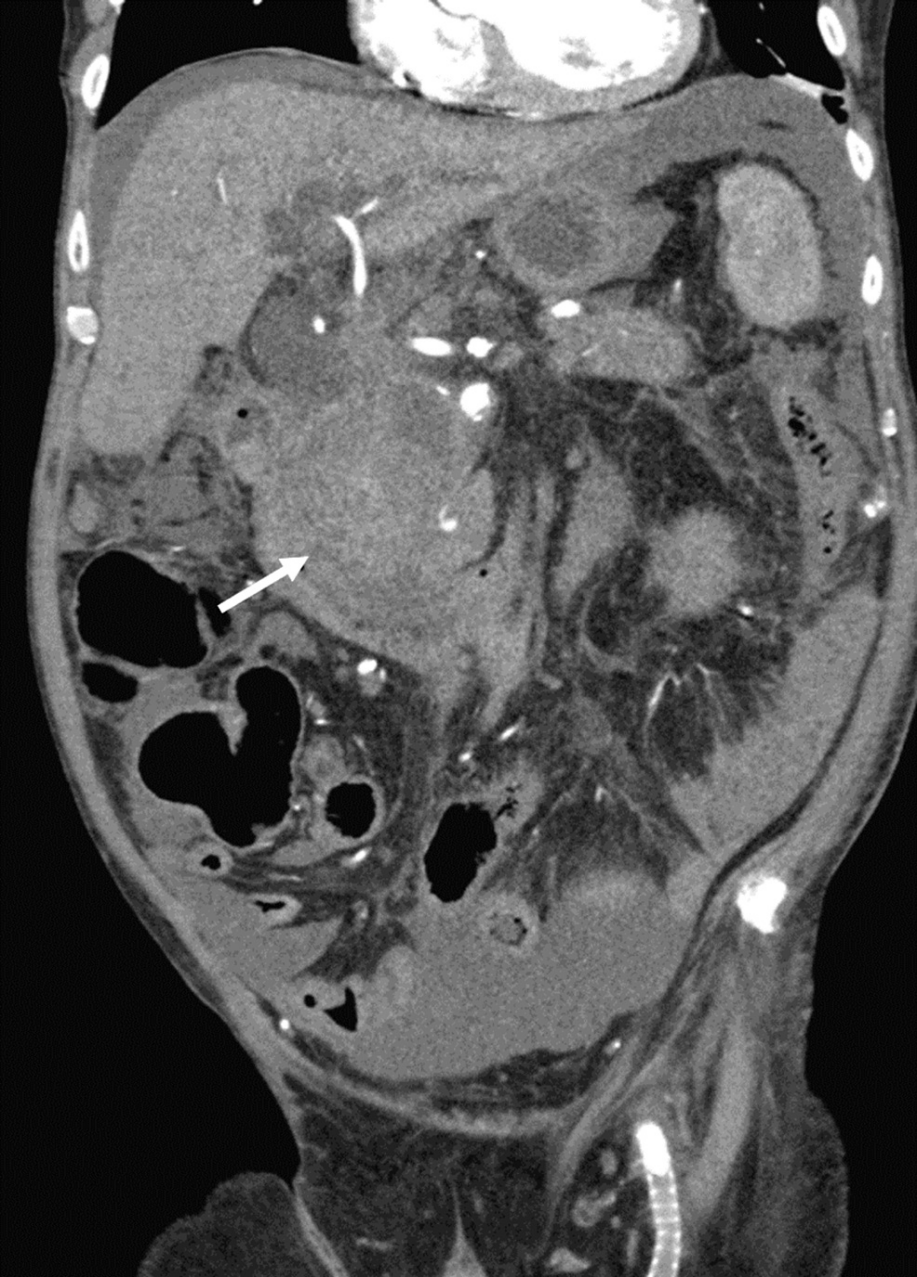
Non-contrasted CT scan of the abdomen and pelvis (coronal view). The white arrow points at a large, abnormal heterogeneous structure that was found at the level of the head of the pancreas. Due to the mass effect of this structure, there is common bile duct compression and upstream dilatation of the biliary system. Intrahepatic ductal dilatation can be appreciated.
